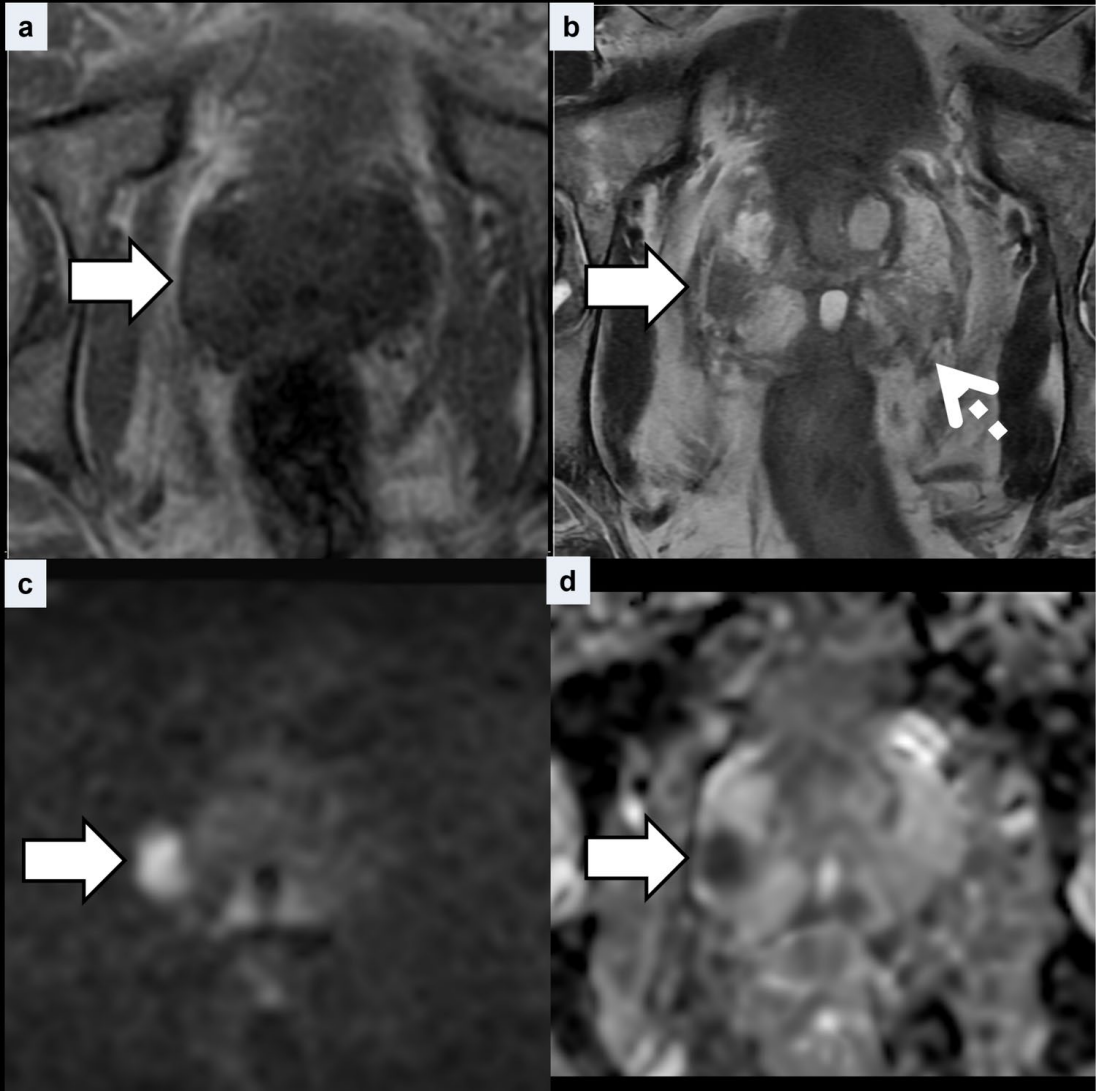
Representative case of prostate carcinoma in a man in his 70s. a Axial T1-weighted image shows a hyperintense nodule in the right peripheral zone (arrow). The lesion-muscle signal intensity ratio, measured using a region of interest on the left obturator muscle, was 1.28. b On the corresponding axial T2-weighted image, the nodular lesion demonstrates hypointensity with a distinct margin (arrow). A wedge-shaped lesion of moderate hypointensity in the left PZ is consistent with chronic prostatitis (dashed arrow). c The lesion demonstrates marked hyperintensity on an axial diffusion-weighted image (arrow). d The corresponding ADC map shows decreased ADC values (0.70 × 10⁻^3^ mm^2^/s)
